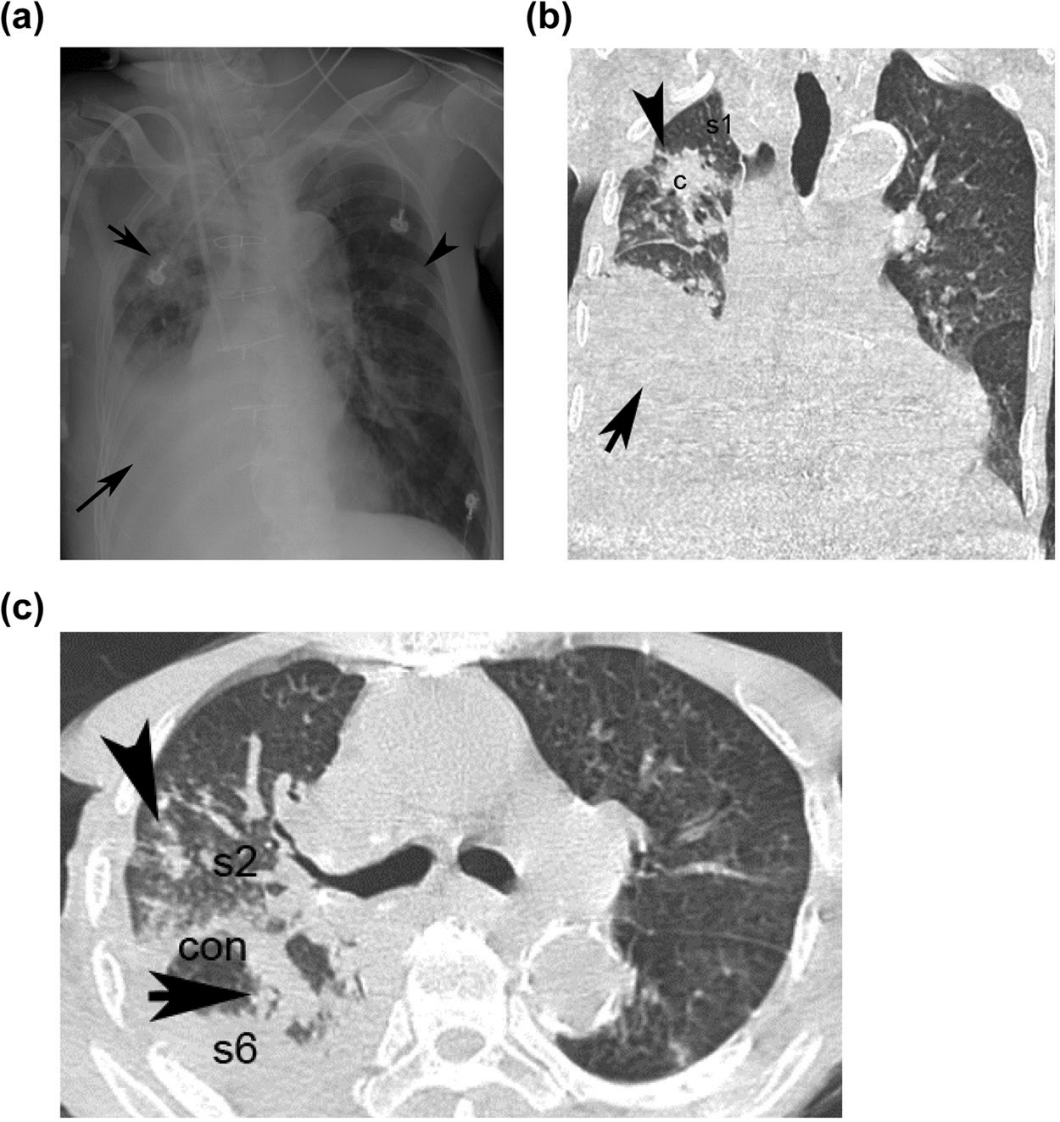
Presentation of an 82-year-male of [aPTB] with acute lung injury presenting as fever (score = 0), dyspnea (score = 0) in HRCT and [CXR + Hypoalbuminemia] model; Hypoalbuminemia (score = 1) in [CXR + Hypoalbuminemia] model. The CXR shows right (right upper black arrowhead)/left (left upper black arrow) upper lung field patch/nodules, (score = 1) and right lower lung field consolidation with pleural effusion (right lower black arrow) (score = 0) (a). The coronal section of HRCT shows clusters of mass of s1 of right upper lobe (right upper black arrowhead) (score = 2), pleural effusion with consolidation (right lower black arrow) of right lower lobe (b); The transverse section of HRCT shows clusters nodules in s2 (score = 2) of the right upper lobe (right black arrow) and consolidation in s6 of the right lower lobe (right black arrowhead) (score = 1) (c). The total score in [CXR (score1) + Hypoalbuminemia (score1)] model is 2; total score in the HRCT model is 3 [clusters of mass/nodules in s1/s2 of right upper lobe (score2) + consolidation in s6 of right lower lobe (score1)]. c = cluster nodules/mass; cav = cavitation; s1 = apical segment; s2 = posterior segment right upper lobe; s1 + s2 = apico-posterior segment left upper lobe; s6 = superior segment of right or left lower lobe.
